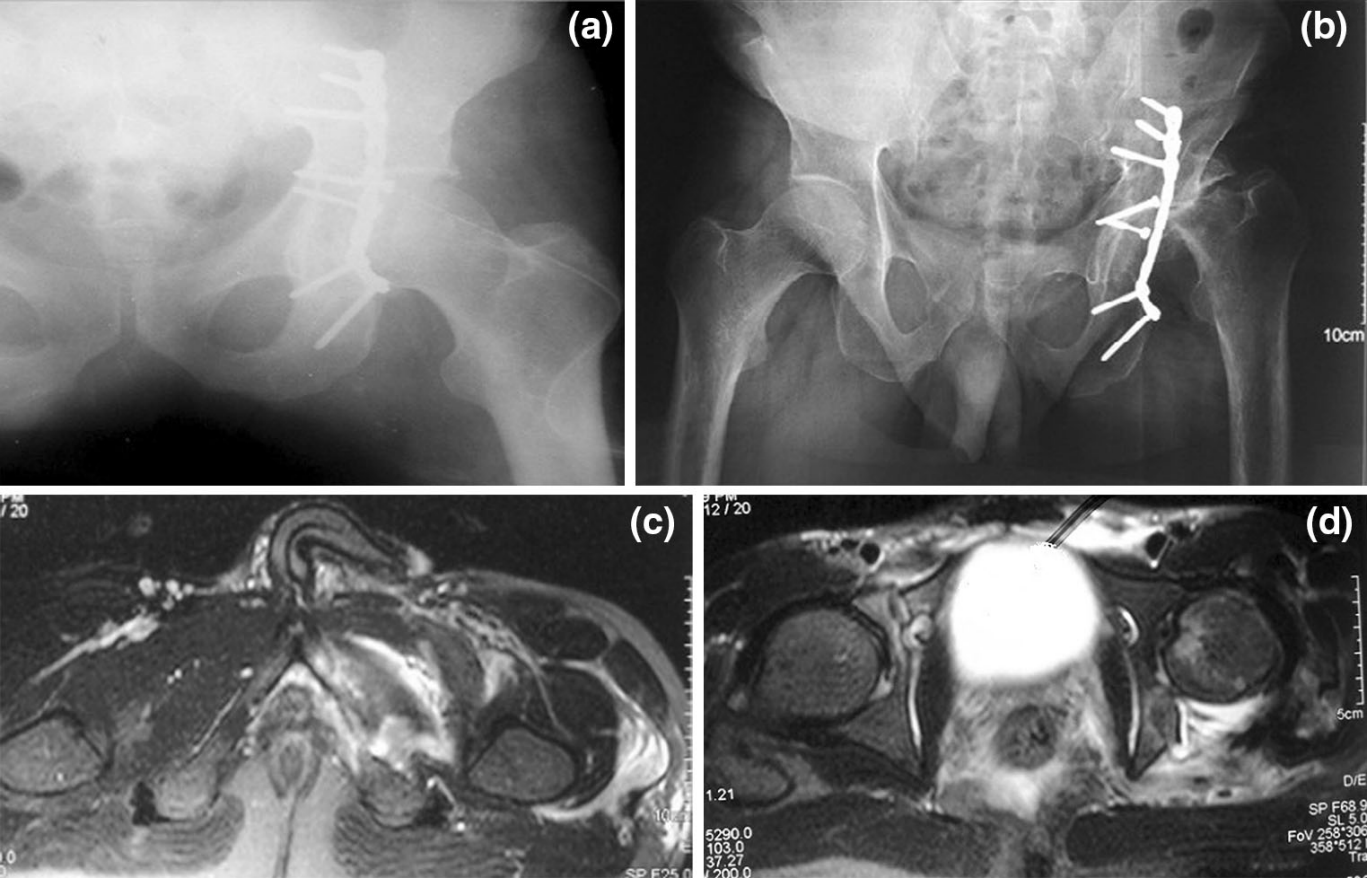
Imaging of case 6 with posterior wall fracture. a 1-month post-operative antero-posterior radiograph showing operative fixation of the fracture with buttress reconstruction plate and normal femoral head; b 3-month post-operative radiograph showing avascular necrosis of femoral head with resorption; c pre-operative MRI of the patient had shown a complete tear of obturator externus; and d a complete tear of piriformis
